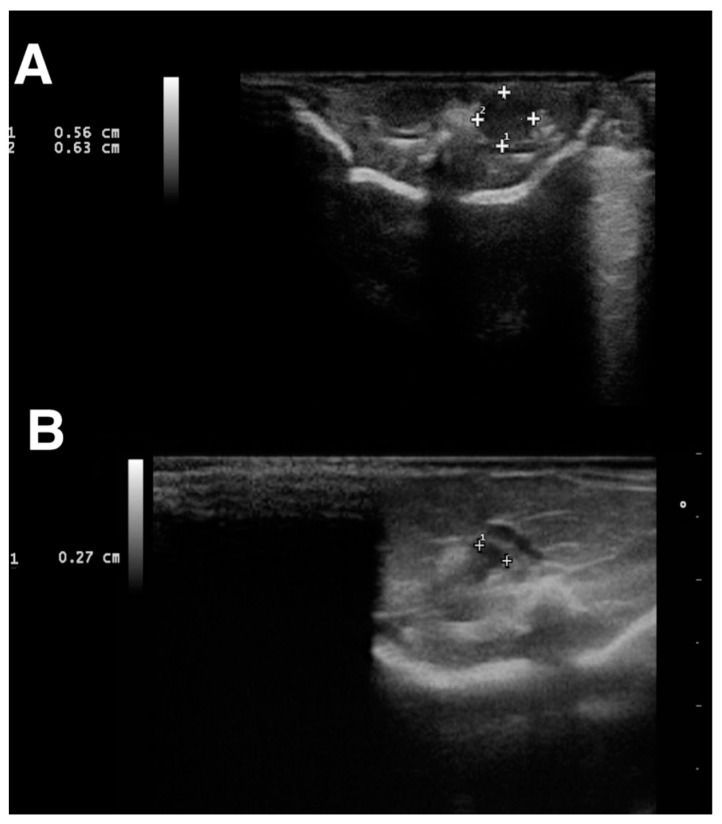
Ultrasonographic examination was performed 8 months after the contrast radiographic study (T3). 1: longitudinal diameter. 2: Transversal diameter (A) Transverse plane, dorsal recumbency, dorsocaudal coelom: 0.6 cm c.ca., ovoid in shape and homogeneous in echogenicity organs were identified as testicles in 15 lizards. (B) Axial plane, dorsal recumbency, dorsocaudal coelom (left side): ovoid organs characterized by heterogeneous echogenicity due to the presence of numerous 2 mm c.ca anechoic follicles on the organ’s surface were identified as testicles in 8 lizards. 1: transversal diameter.
